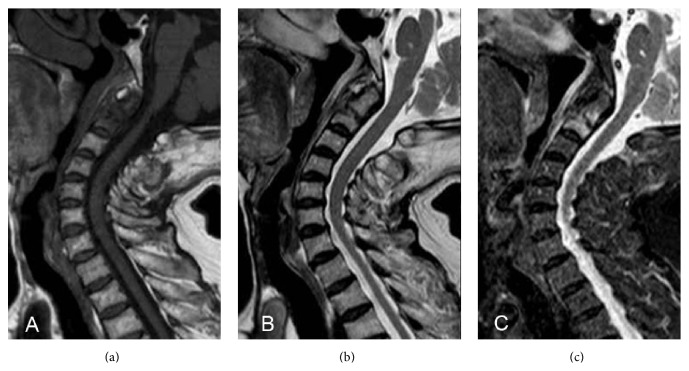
Magnetic resonance imaging demonstrated overt intensity changes of C2, which was low intensity on both (a) T1- and (b) T2-weighted images but high intensity on STIR (c) images. Spinal cord involvement was not evident.
